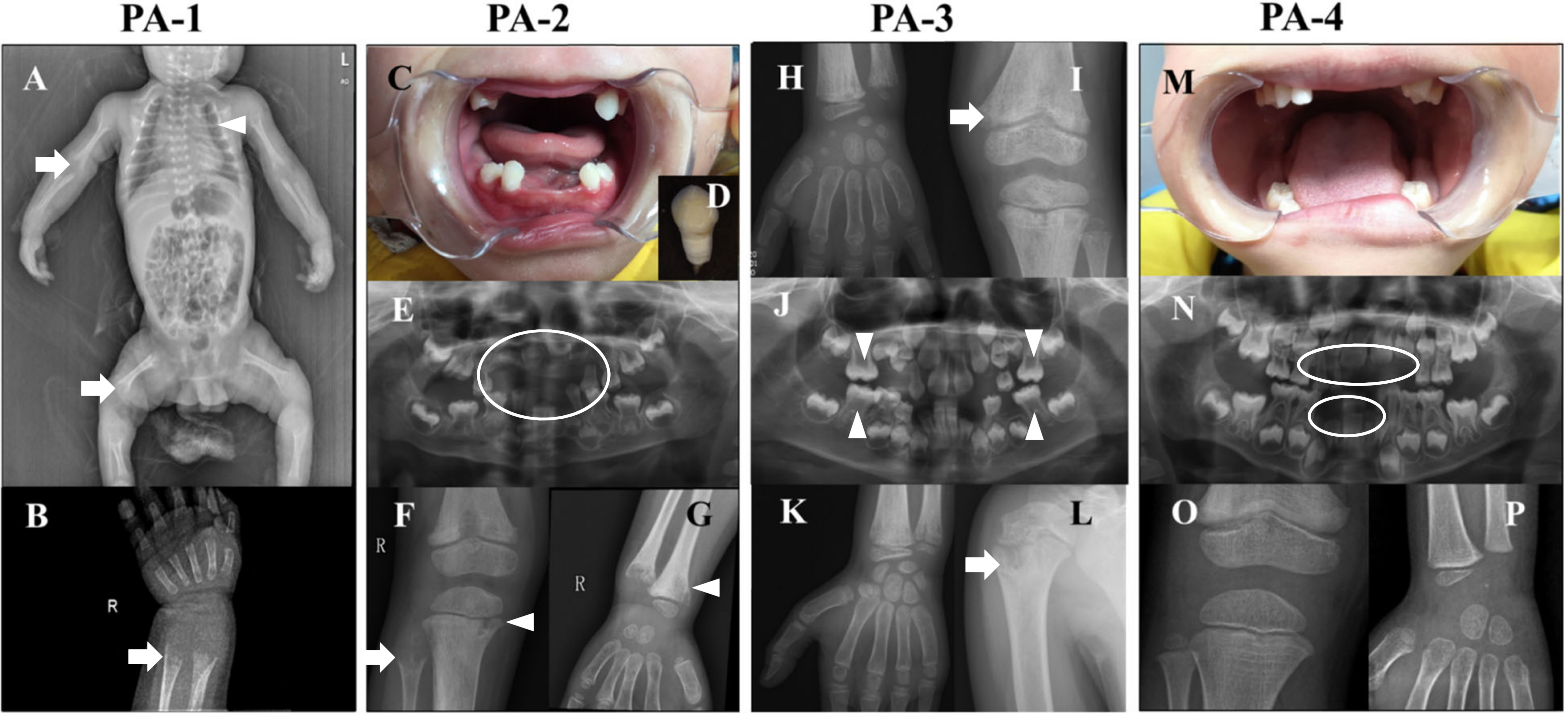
Photos and X-rays of teeth and bones in 4 HPP children with HPP. a & b X-rays show general hypomineralization, gracile ribs (arrowhead), small clavicles, shortened long bones and flared metaphysis (arrow) in patient 1 (PA-1) at 2-month-old; c, d & e Premature loss of deciduous tooth (circle) in Patient 2 (PA-2) at 2-year-old; f & g X-rays show flared metaphysis (arrow), and bone destruction (arrowhead) in PA-2; h & i X-ray shows hypomineralization (arrow) in PA-3 at 4-year-old; j Panoramic X-ray in PA-3 at 8-year-old shows decreased mineralization of the alveolar bone, enlarged pulp chambers (arrowhead) and alveolar bone resorption; k & l Bone destruction in the proximal humerus (arrow) of PA-3 at 8-year-old; m & n Premature loss of deciduous tooth (circle) in Patient 4 (PA-4) at 4-year-old; o & p X-ray didn’t show significant bone abnormality
